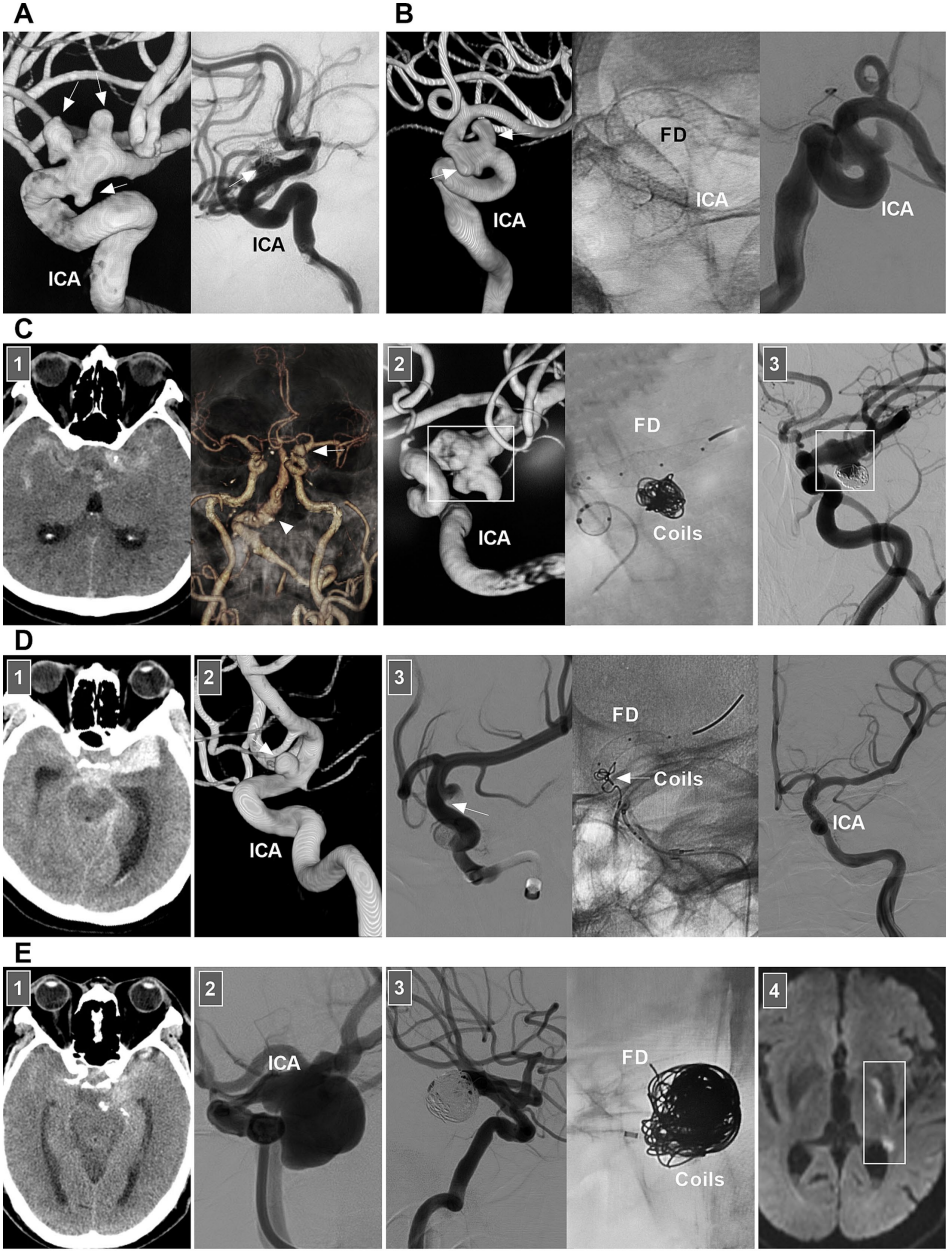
EVT of supraclinoid ICA dissections. (A) Left panel: DSA showing multiple dissecting aneurysms (arrows) on supraclinoid ICA. Right panel: one-year follow-up DSA showing the embolization was incomplete after traditional stent-assisted coiling (arrow). (B) Left panel: DSA showing multiple dissecting aneurysms (arrows) on supraclinoid ICA. Middle panel: X-ray image showing the FD in supraclinoid ICA. Right panel: DSA showing the ICA reconstruction. (C) Panel 1: CT (left panel) showing SAH; CTA (right panel) showing a PcomA aneurysm (arrow) and vertebrobasilar dilatation (arrowhead). Panel 2: DSA (left panel) showing a supraclinoid ICA dissection with a PcomA aneurysm (frame); X-ray image (right panel) showing FD deployment with coiling to treat the dissection. Panel 3: Six-month follow-up DSA showing that the supraclinoid ICA was reconstructed (frame). (D) Panel 1: CT showing SAH. Panel 2: DSA showing a supraclinoid ICA aneurysm (arrow). Panel 3: DSA (left panel) showing that the aneurysm was dissecting, the arrow indicated the entry of the dissection; X-ray image (middle panel) showing the entry of the dissection was coiled (arrow) under the assistance of FD deployment; DSA (right panel) showing the dissection cannot be seen. (E) Panel 1: CT showing SAH. Panel 2: DSA showing a large supraclinoid ICA dissecting aneurysm (arrow). Panel 3: DSA (left panel) showing that the aneurysm was coiled, X-ray image (right panel) showing the coiling under the assistance of FD deployment. Panel 4: Diffuse weighted image of magnetic resonance showing acute ischemia (frame) of the region supplied by anterior choroidal artery. CT, computed tomography; CTA, CT angiography; DSA, digital subtraction angiography; EVT, endovascular treatment; FD, flow diverter; ICA, internal carotid artery; PcomA, posterior communicating artery; SAH, subarachnoid hemorrhage.
